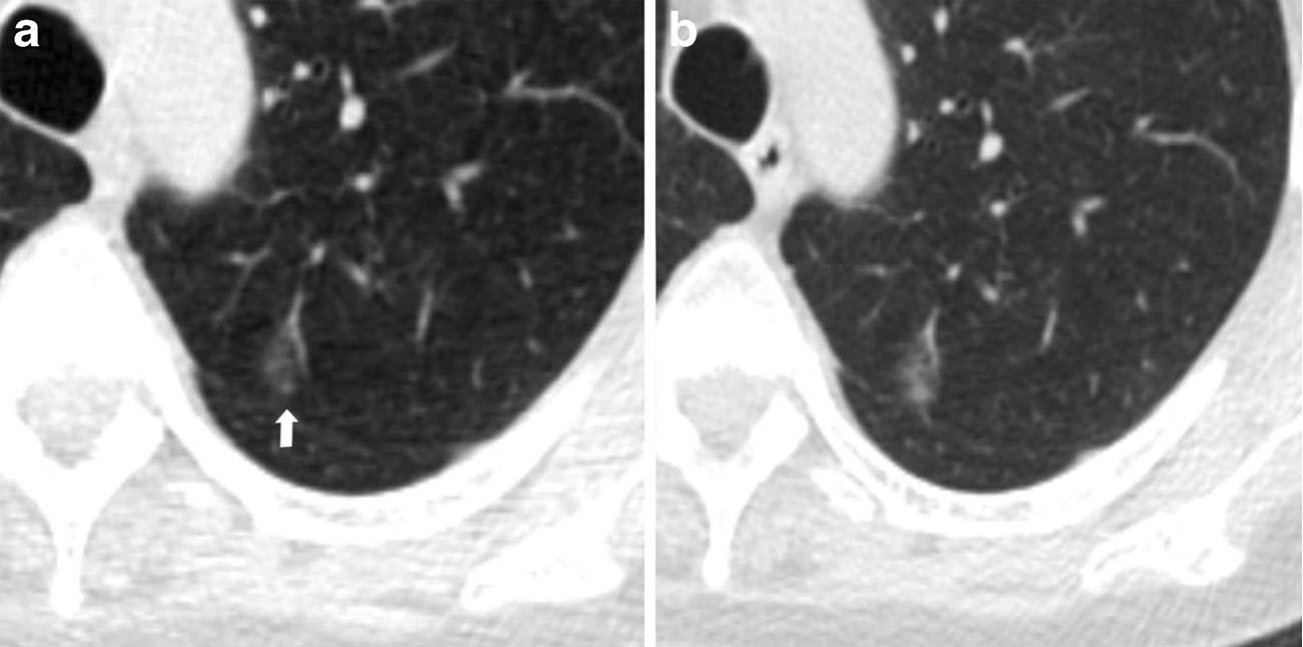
Progression at the 1-year interval of a non-solid nodule in a 66-year-old woman. (a) Low-dose CT section shows a 12-mm nodule with ground-glass attenuation of the upper left lobe (arrow). (b) CT scan obtained at annual repeat LDCT demonstrates a substantial stability in size but with increased density, that indicates an increased risk for malignancy. The lesion was surgically removed with a diagnosis of lung adenocarcinoma. Sub-solid nodules that show an increment in attenuation should be considered suspicious for malignancy
